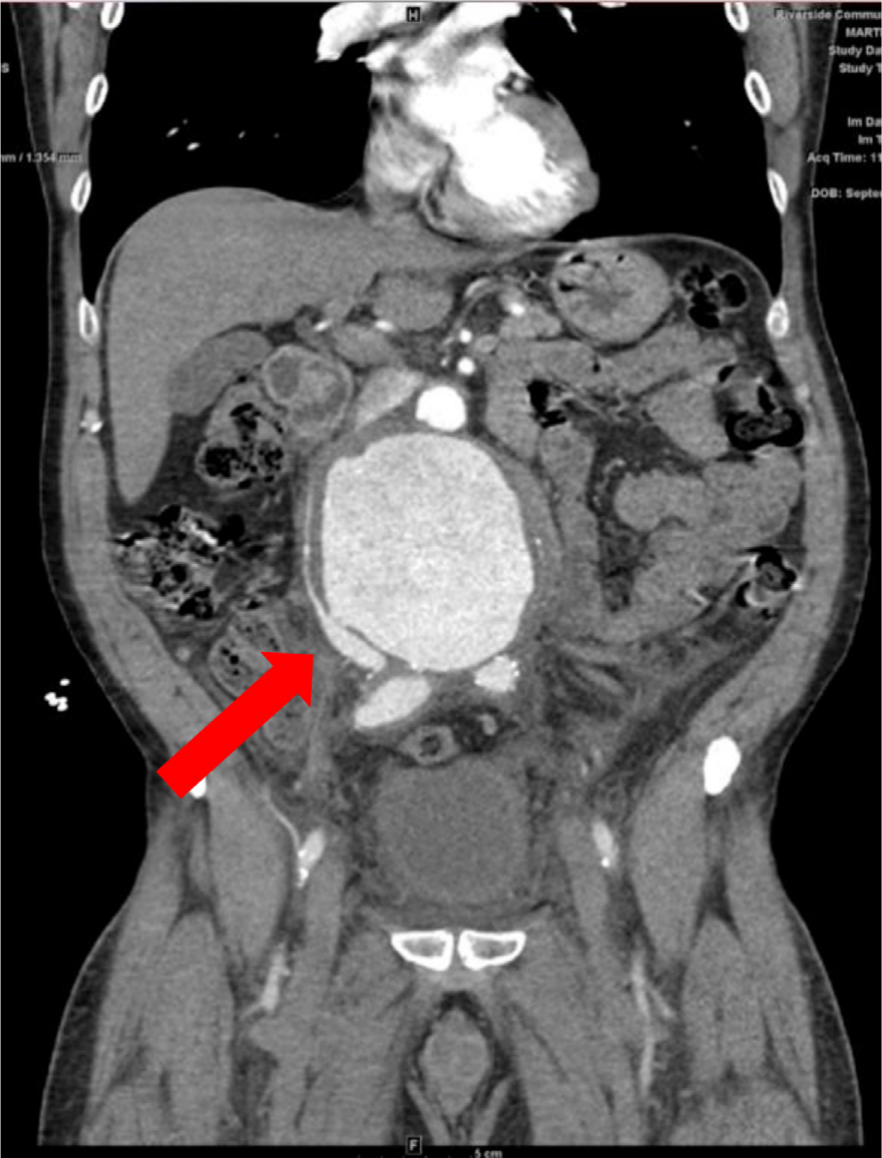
CTA demonstrates a contained rupture of the thickened and fibrotic aneurysmal wall into the adjacent vena cava.
